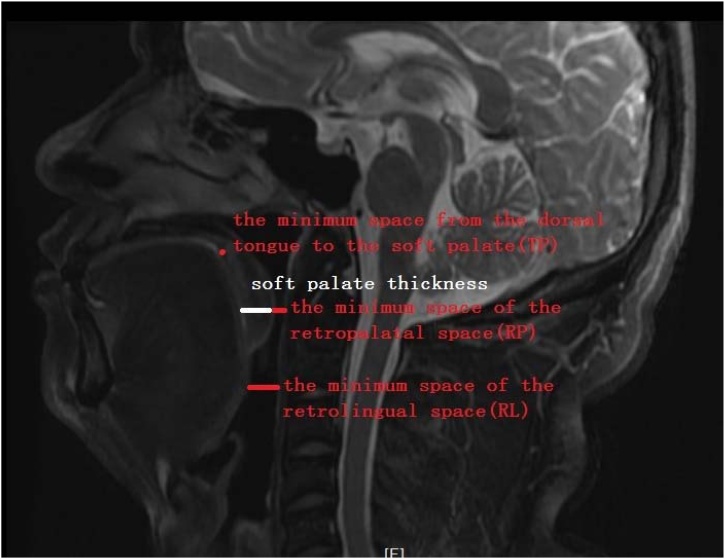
Measurement method of the Pharyngeal MRI.
